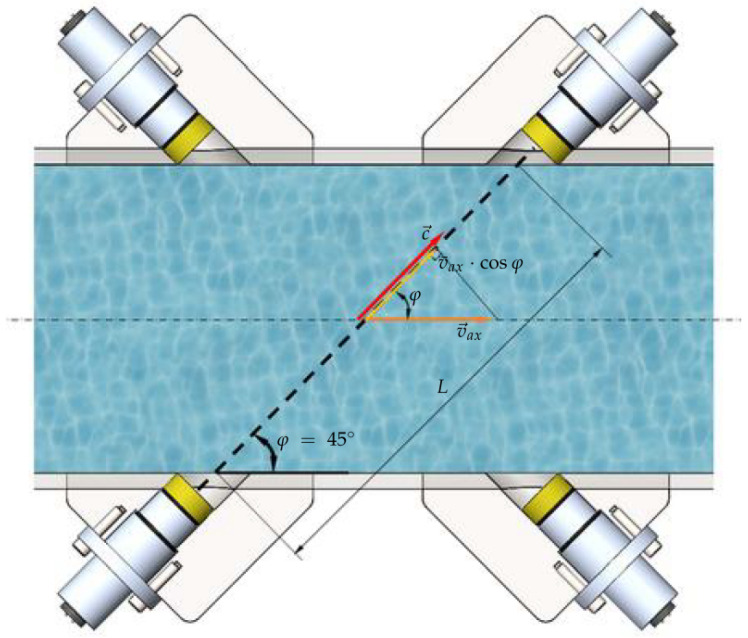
Measurement of time-of-flight in a conduit using multiple acoustic trajectories of ultrasonic transducers.
